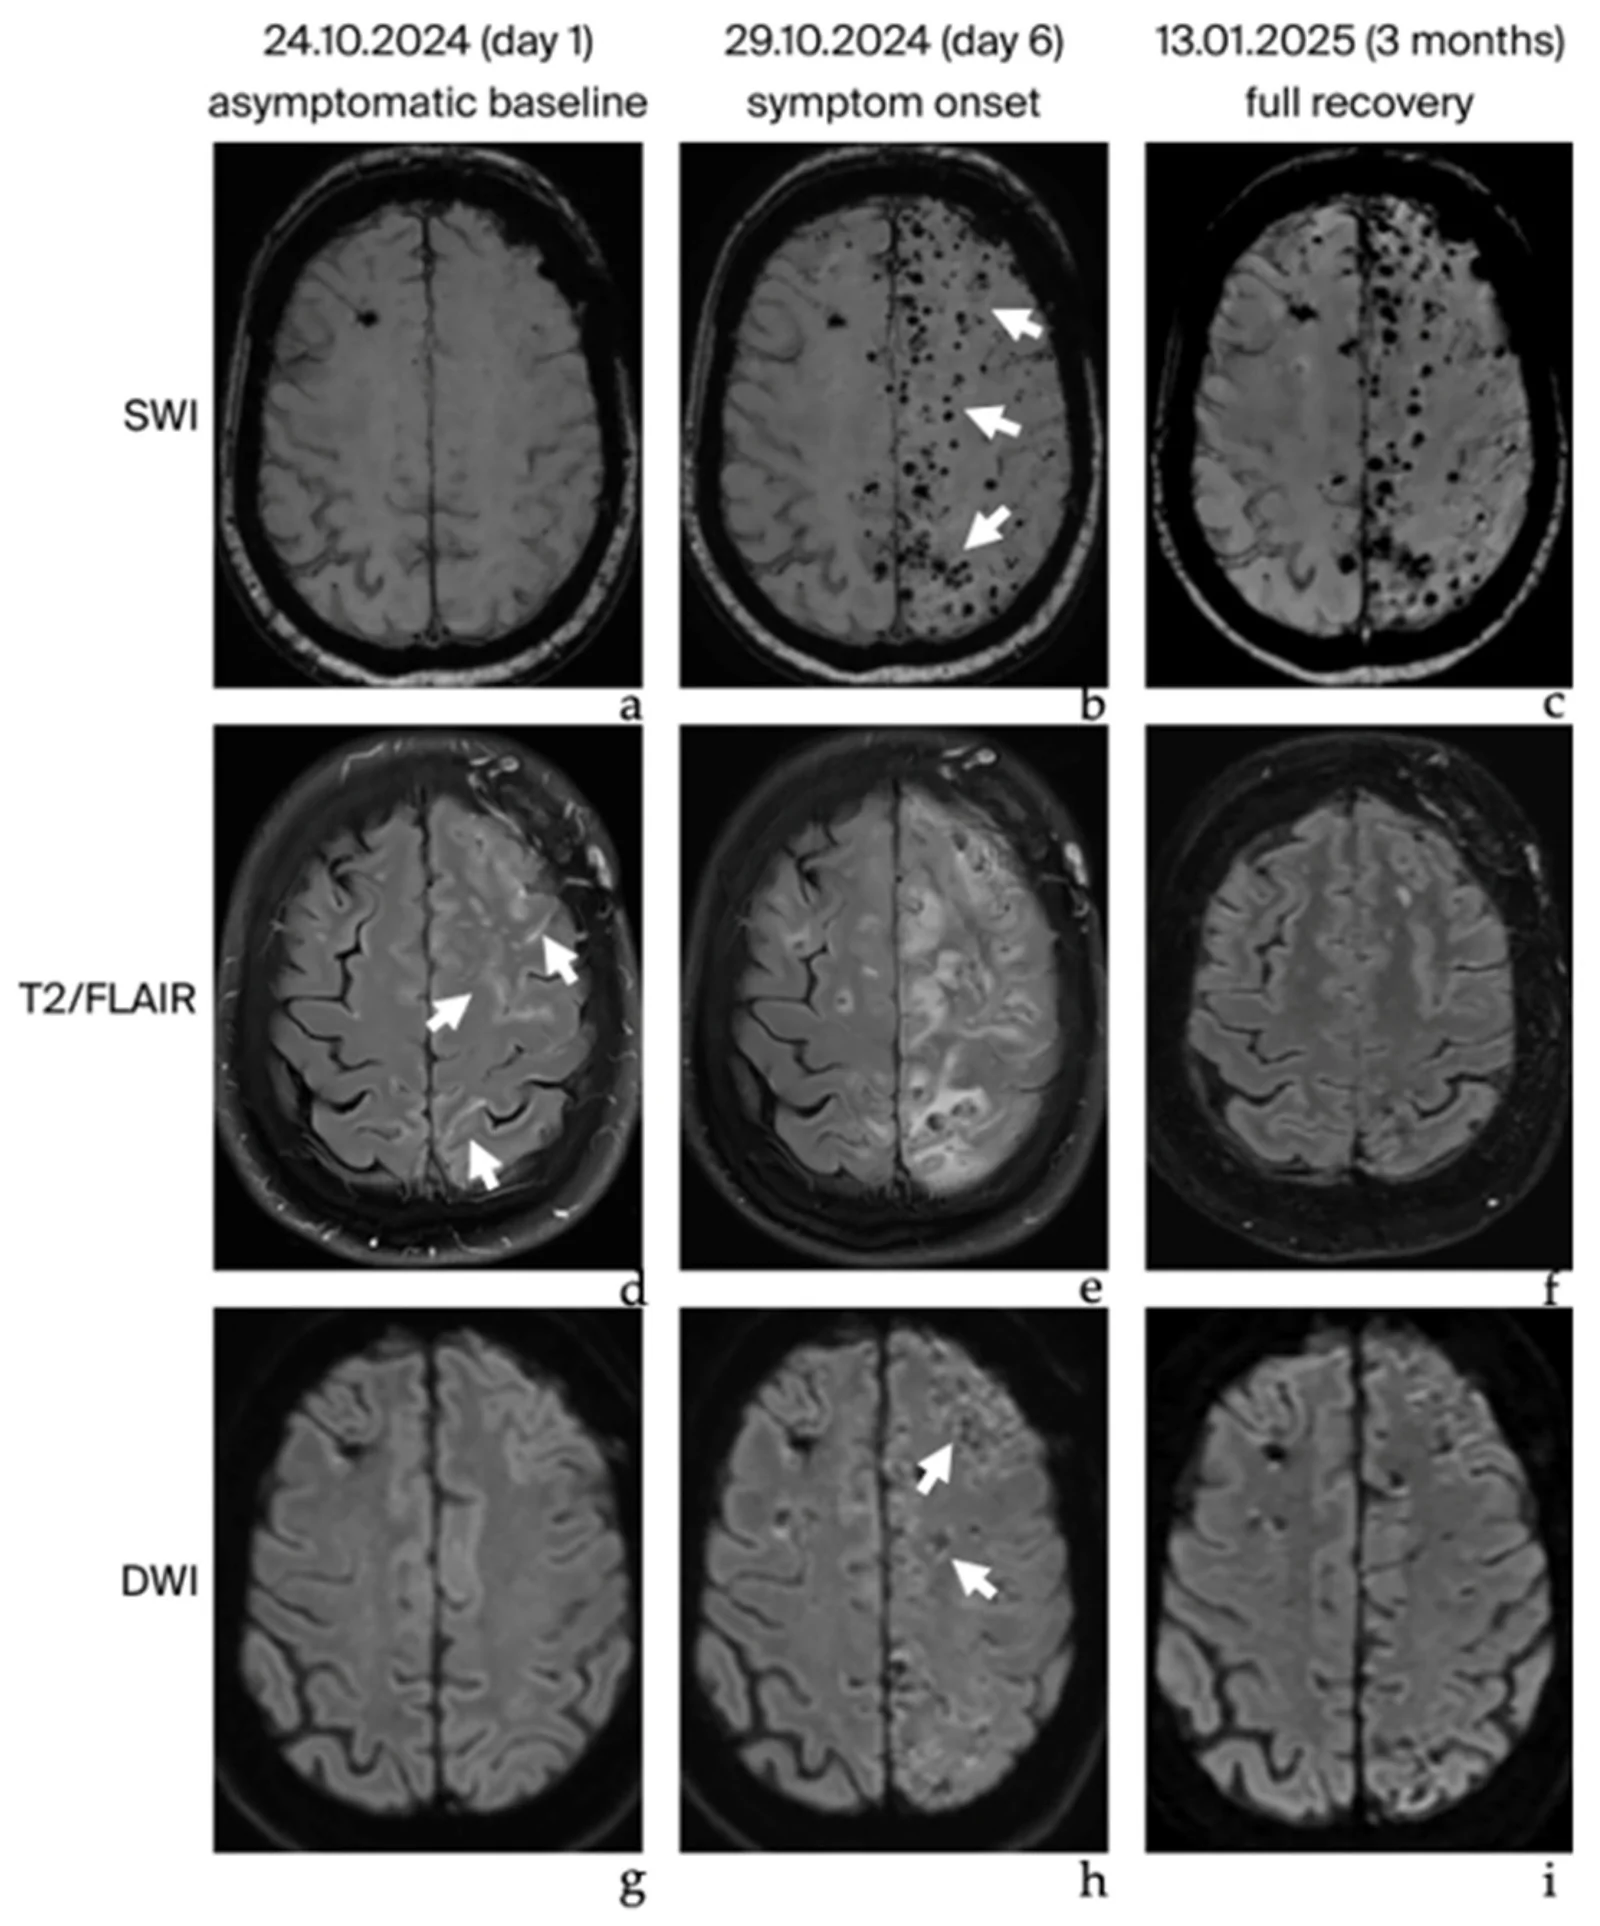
Timeline of brain magnetic resonance imaging findings of late-onset CIE. (a–c): Susceptibility weighted imaging (SWI) demonstrating multiple cerebral microbleeds at symptom onset (Image (b)—arrows). (d–f): T2-weighted fluid attenuated inversion recovery (FLAIR) imaging showing mild leptomeningeal effusion on day 1 (Image (d)—arrows), progression at symptom onset (Image (e)), and complete resolution at the 3-month follow-up (Image (f)). (g–i): Diffusion-weighted imaging (DWI) demonstrating patchy cortical and subcortical signal abnormalities at symptom onset (Image (h)—arrows) with regression at the 3-month follow-up (Image (i)).
